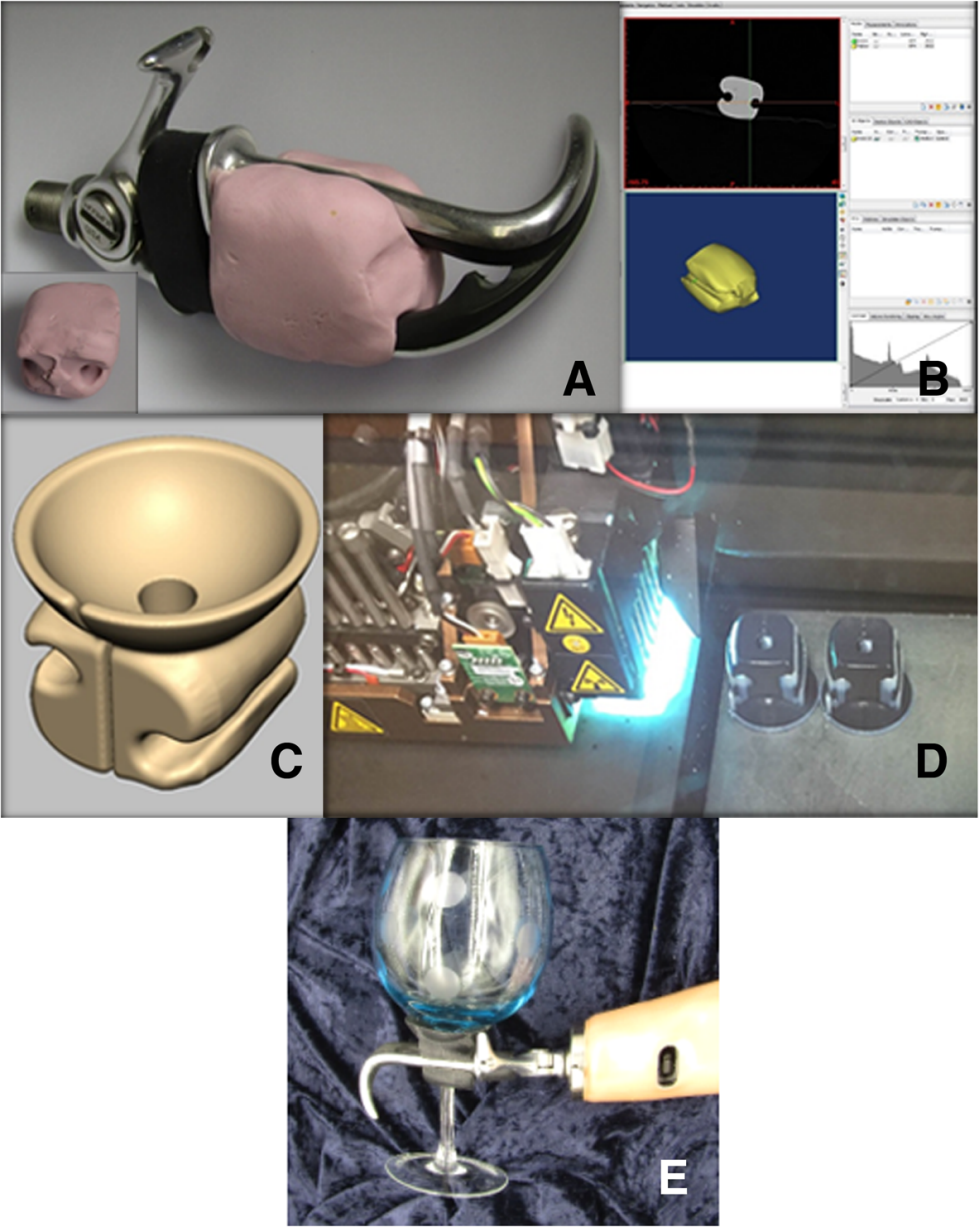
Wine Glass Holder. a Lab putty was used to make impression of the space between the prosthetic hook. b Putty impression was scanned using computed tomography (CT) at 5 mm slice increment and then reconstructed. STL was exported. c The cup, hole, and slit added to the design. d Device was 3D printed using multi-material jetting machine utilizing flexible material. e Prosthetic attachment enables an amputee to enjoy wine again
